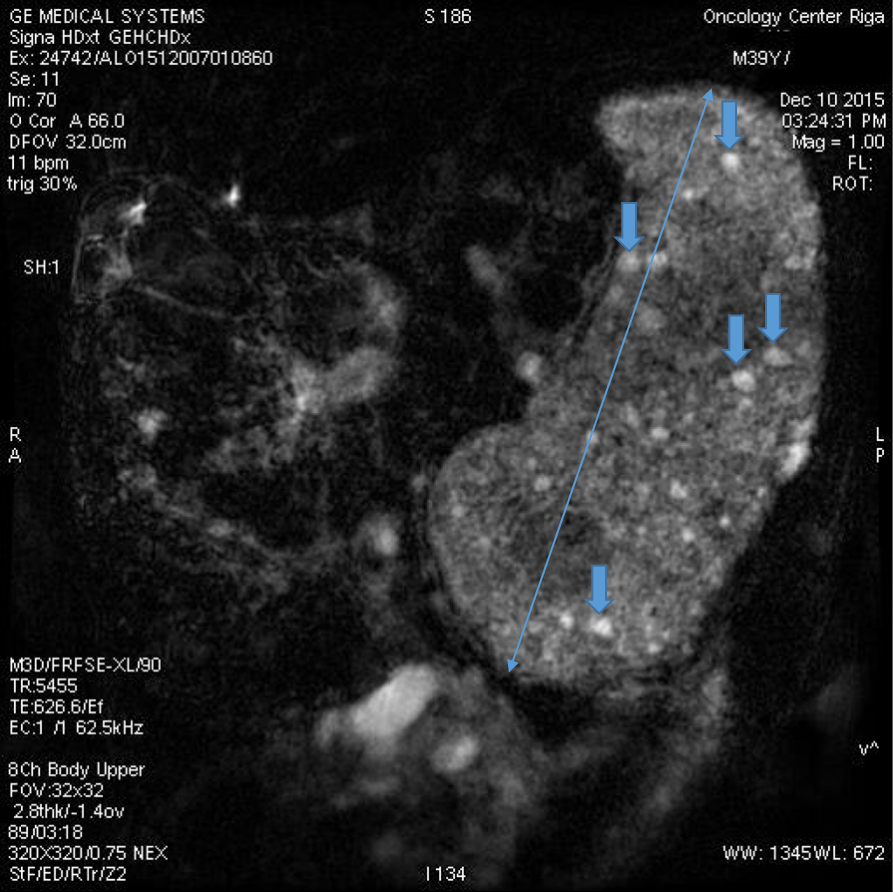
Magnetic resonance imaging (MRI) showing splenomegaly with multiple hemangiomas (*arrows*)
